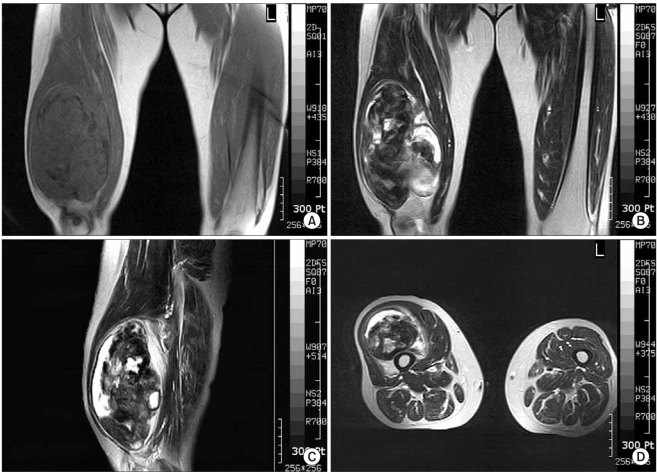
On MRI, the tumor is a 17 × 11 × 9 cm sized mass located under the quadriceps muscle. The tumor matrix is partially calcified and relatively well defined one having irregular low signal intensity in the T1 weighted image (A) and heterogeneous low signal and high signal in the T2 weighted image (B-D).
